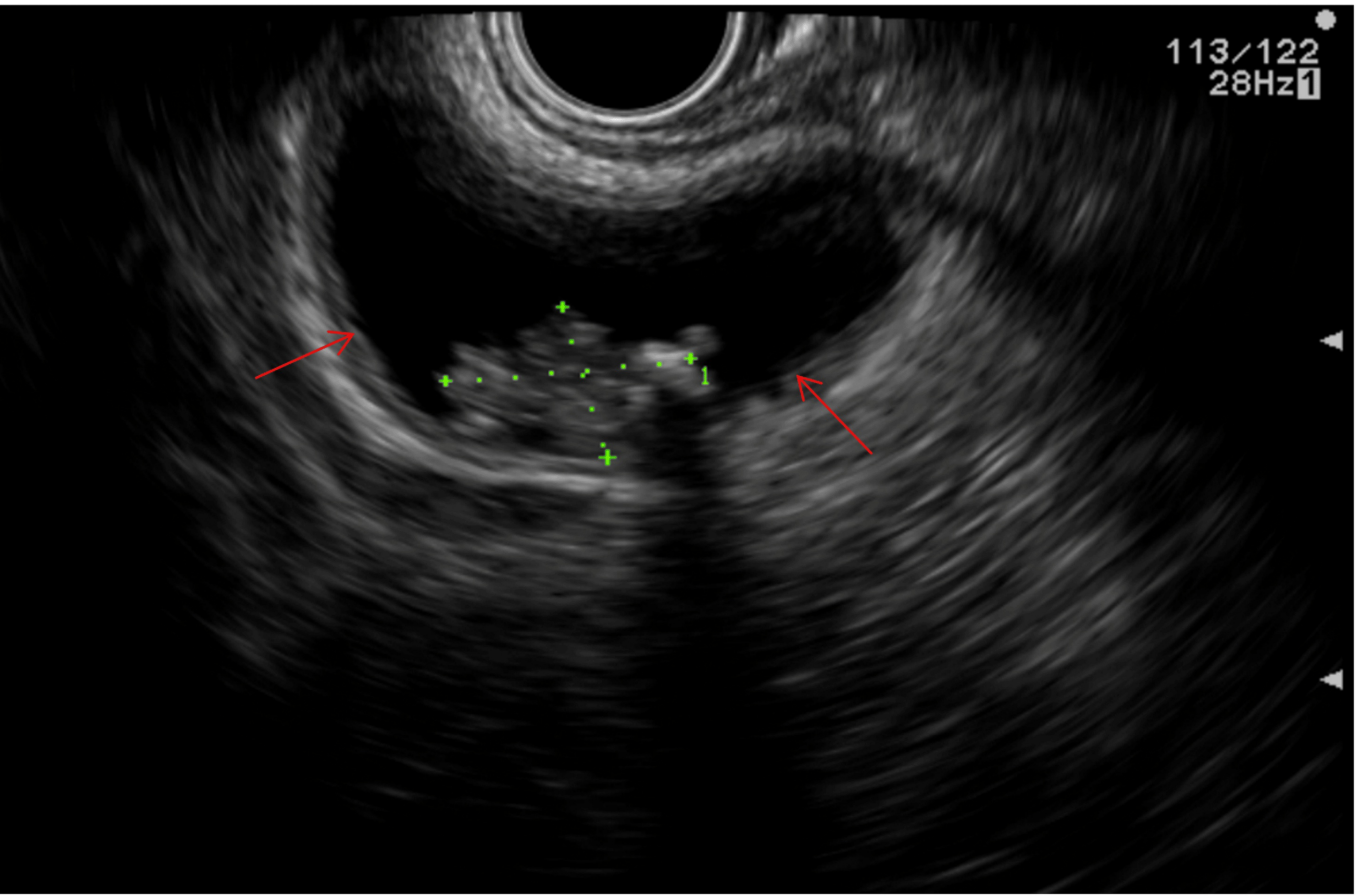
Endoscopic ultrasound (EUS) images showing a large cystic lesion in the head of the pancreas measuring 5 × 6 cm (red arrows) with features suggestive of a cystic neoplasm.
